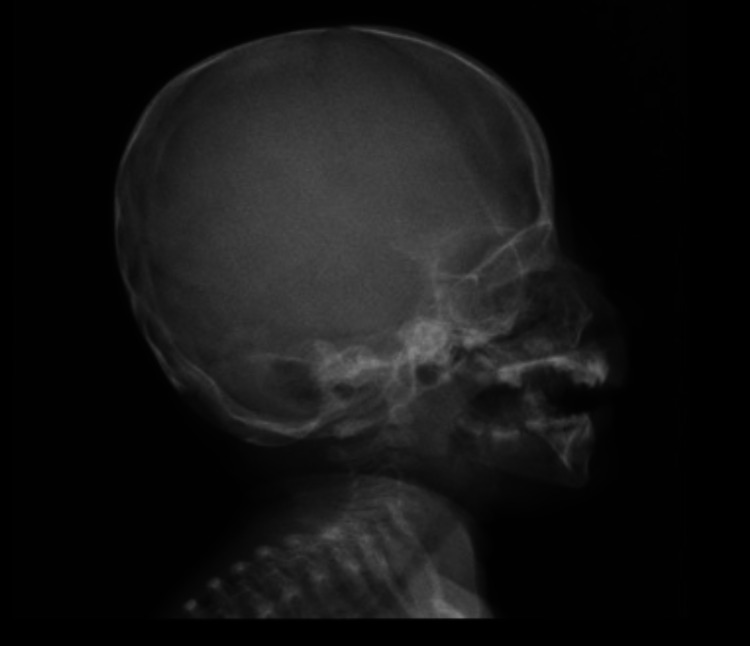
X-ray of the head: lateral view showing normal skull shape with no deformity; however, it is smaller in size for her age.
